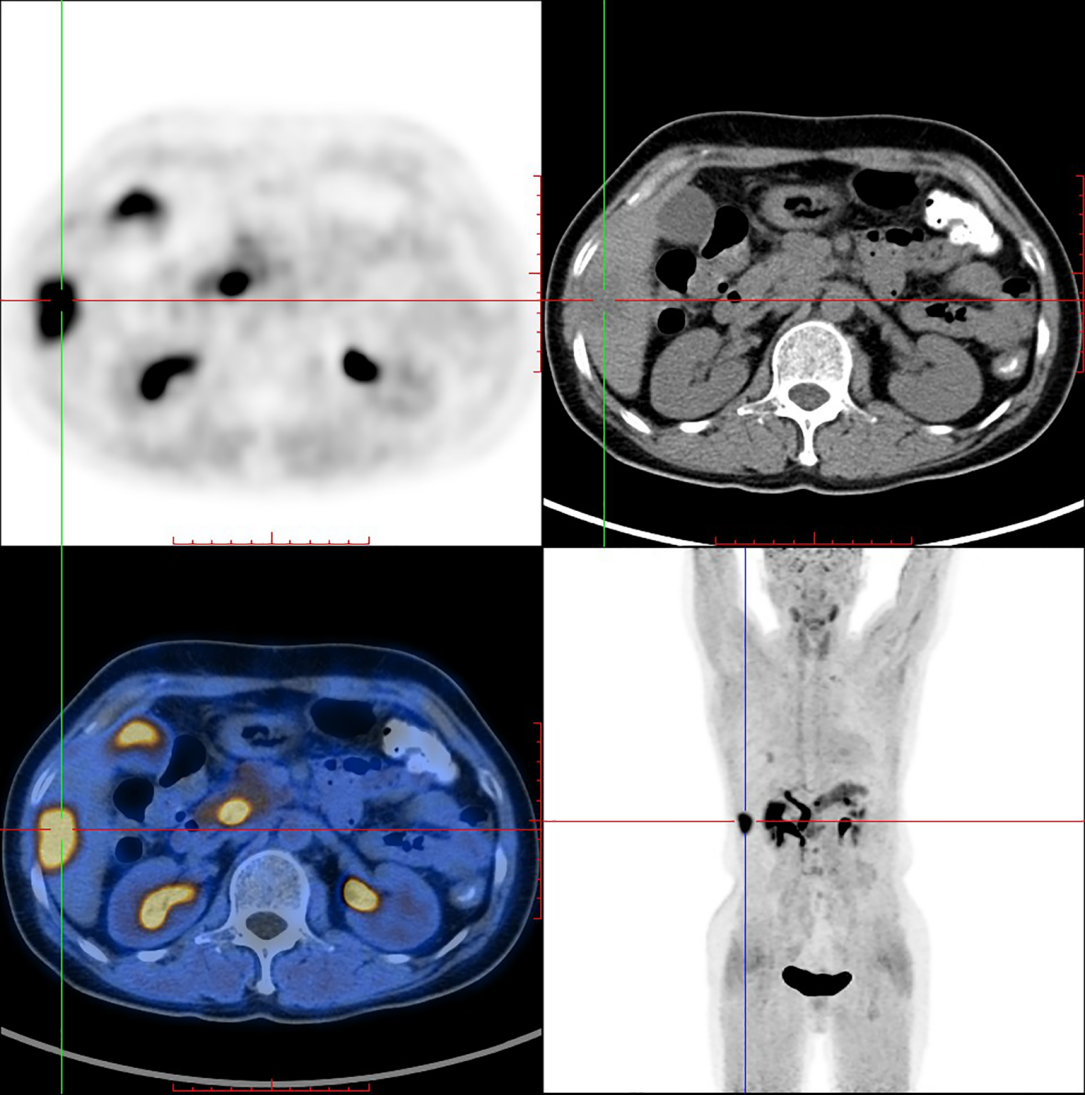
[^18^F]AlF-NOTA-FAPI-04 PET/CT imaging of a female IDC patient (54 y).
